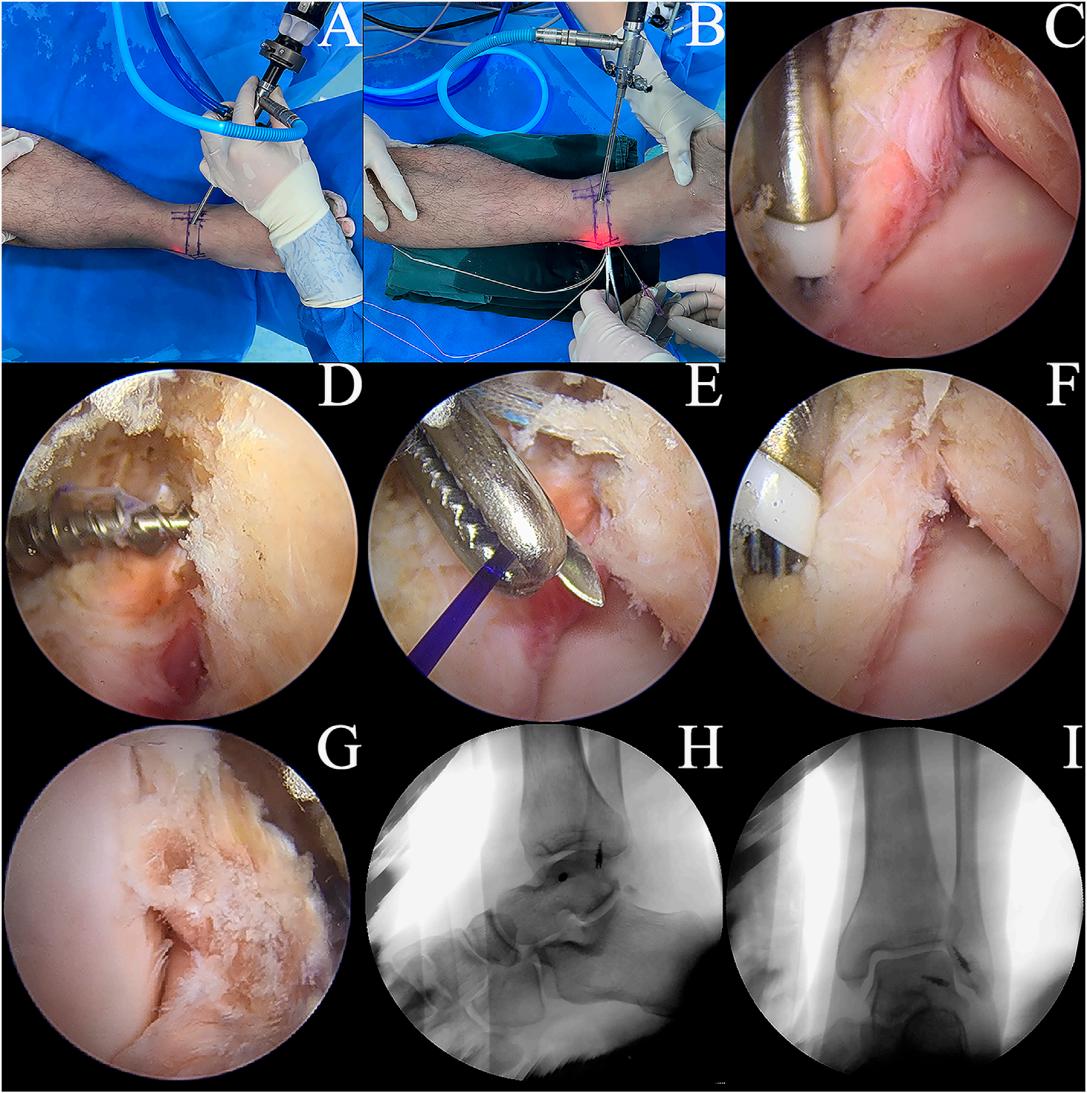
Model development. (A,B) ankle arthroscopic manipulation. (C) A plasma electric knife was used to cut the ATFL at the fibula attachment end of the ATFL. (D) implantation of a metal suture anchor at the attachment end of the talus. (E) suturing of the ATFL and the inferior extensor retinaculum. (F) application of the Broström-Gould procedure to the repaired ATFL. (G) the ATiFL-DF was cut at the attachment end of the tibia using a plasma electric knife and immediately rotated downward and fixed to the talar neck by transposition of the metal suture anchor. (H,I) postoperative lateral and orthopantomogram of the ankle.
